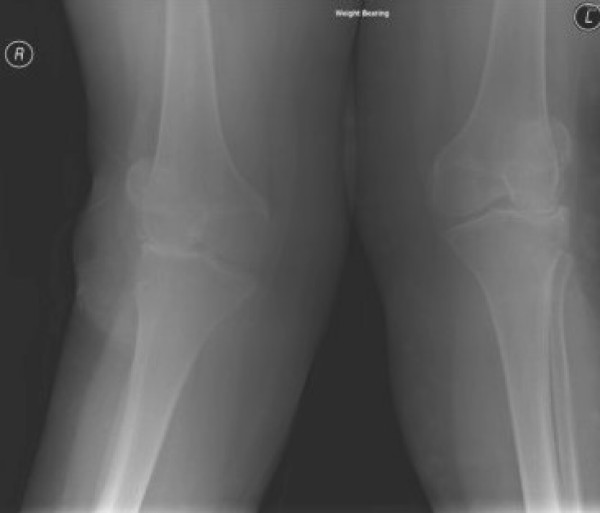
Preoperative weight bearing x-ray of both the knees. Showing severe tricompartmental arthritis and valgus deformity of the right knee.
